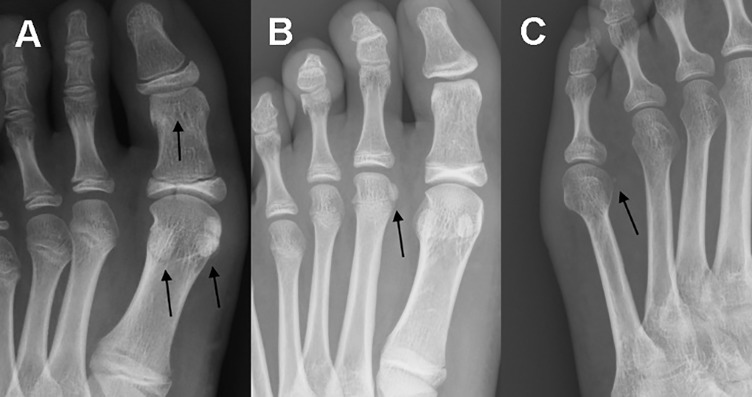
Anteroposterior radiographs of the forefoot of three children, showing the onset appearance of sesamoid bones at the: (A) hallucal metatarsophalangeal and interphalangeal joints; (B) second metatarsophalangeal joint; and (C) fifth metatarsophalangeal joint. Radiographs were obtained from an 8 year old girl (A), a 12 year old boy (B) and a 12 year old girl (C).
